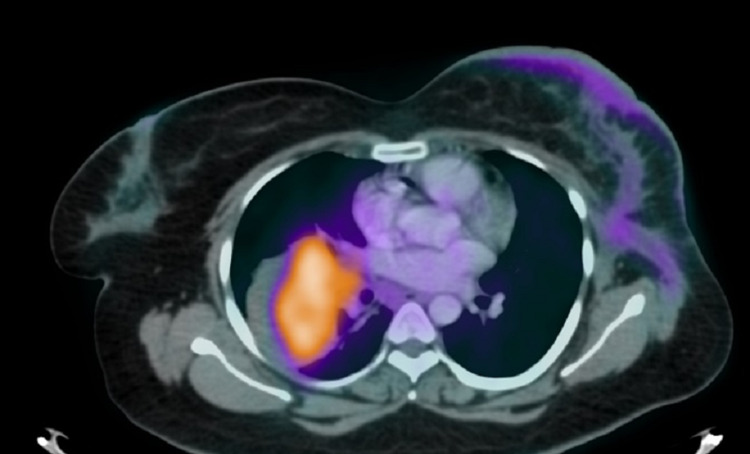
Fused axial slice of FDG PET-CT. A hypermetabolic right lung mass and mild accumulation of FDG in skin thickening overlying the left breast parenchyma. FDG: 18F-fluorodeoxyglucose; PET-CT: positron emission tomography-computed tomography
